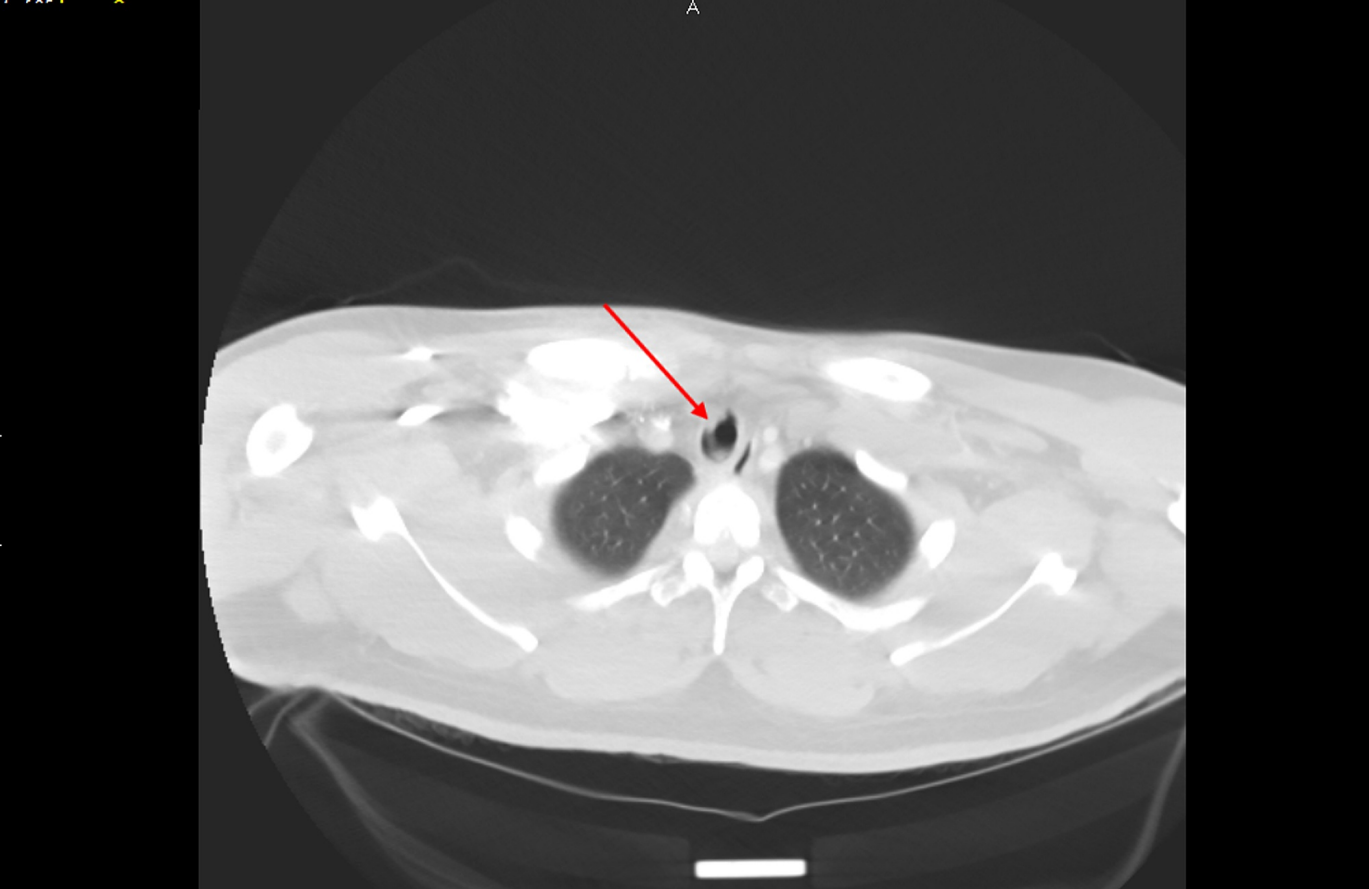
Chest CT scan suggesting the presence of a membranous structure in the trachea (arrow)
